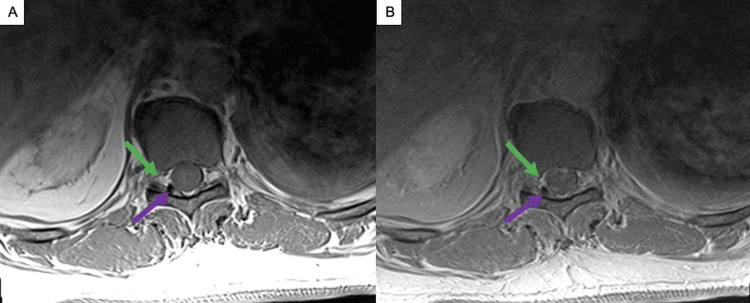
Arterial vascular fistula on thoracic spine MRIs The thoracic spine MRIs shown demonstrate the suggested arterial vascular fistula. (A) is an axial T1-weighted image without contrast and (B) is an axial T1-weighted image with contrast at the T10-11 level, with the green arrows demonstrating prominent areas of enhancement extending into the right T10-T11 foramen suggesting a dural AV fistula. On these axial views, the purple arrows also demonstrate the large, right-sided dorsal flow void seen.
